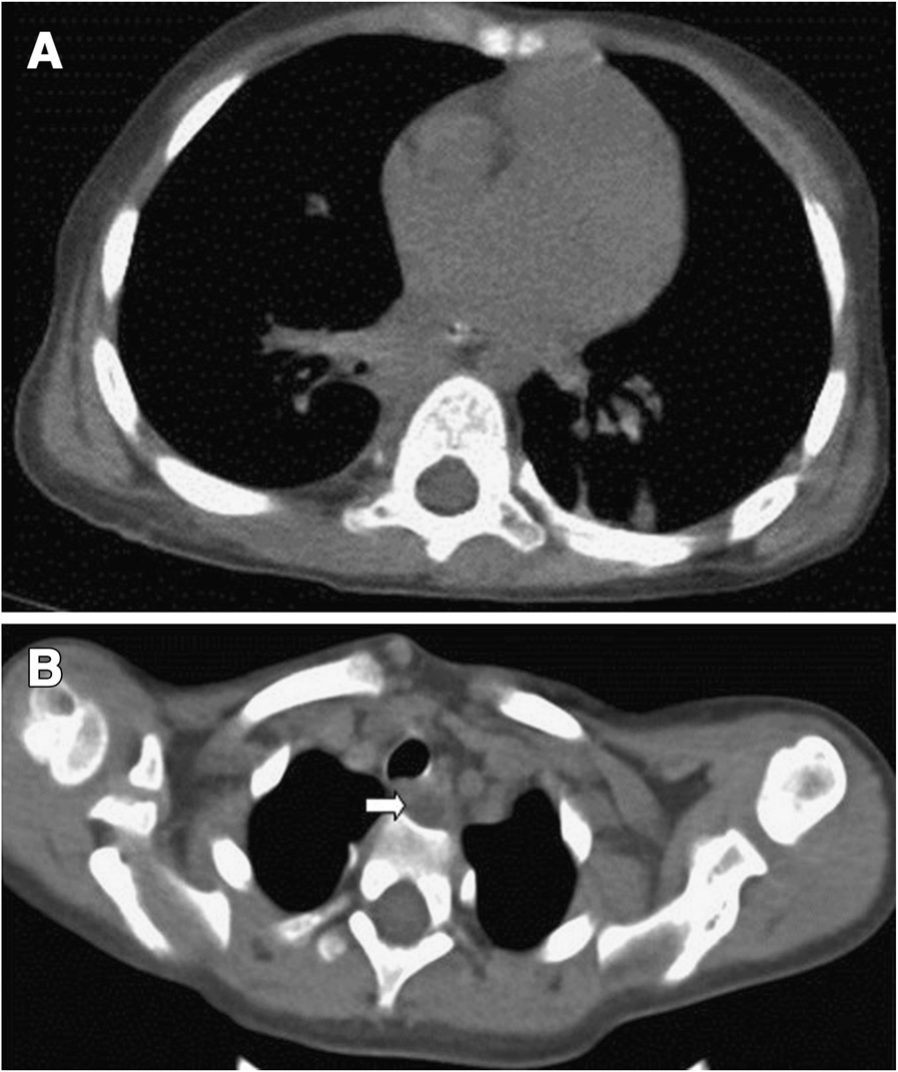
Follow-up chest computed tomography scan. No disease, residual or recurrent, was found for the resected large-sized cyst (A) and no growth of the small-sized cyst was observed (B) (white arrow).
